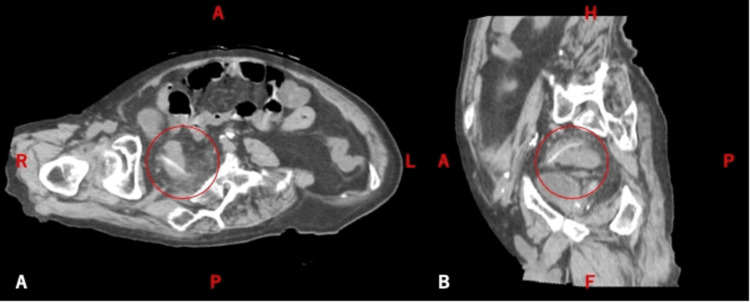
CT reconstruction showing surrounding fat stranding; the tip of the foreign body appears to extend beyond the bowel wall (circle) CT: computed tomography
